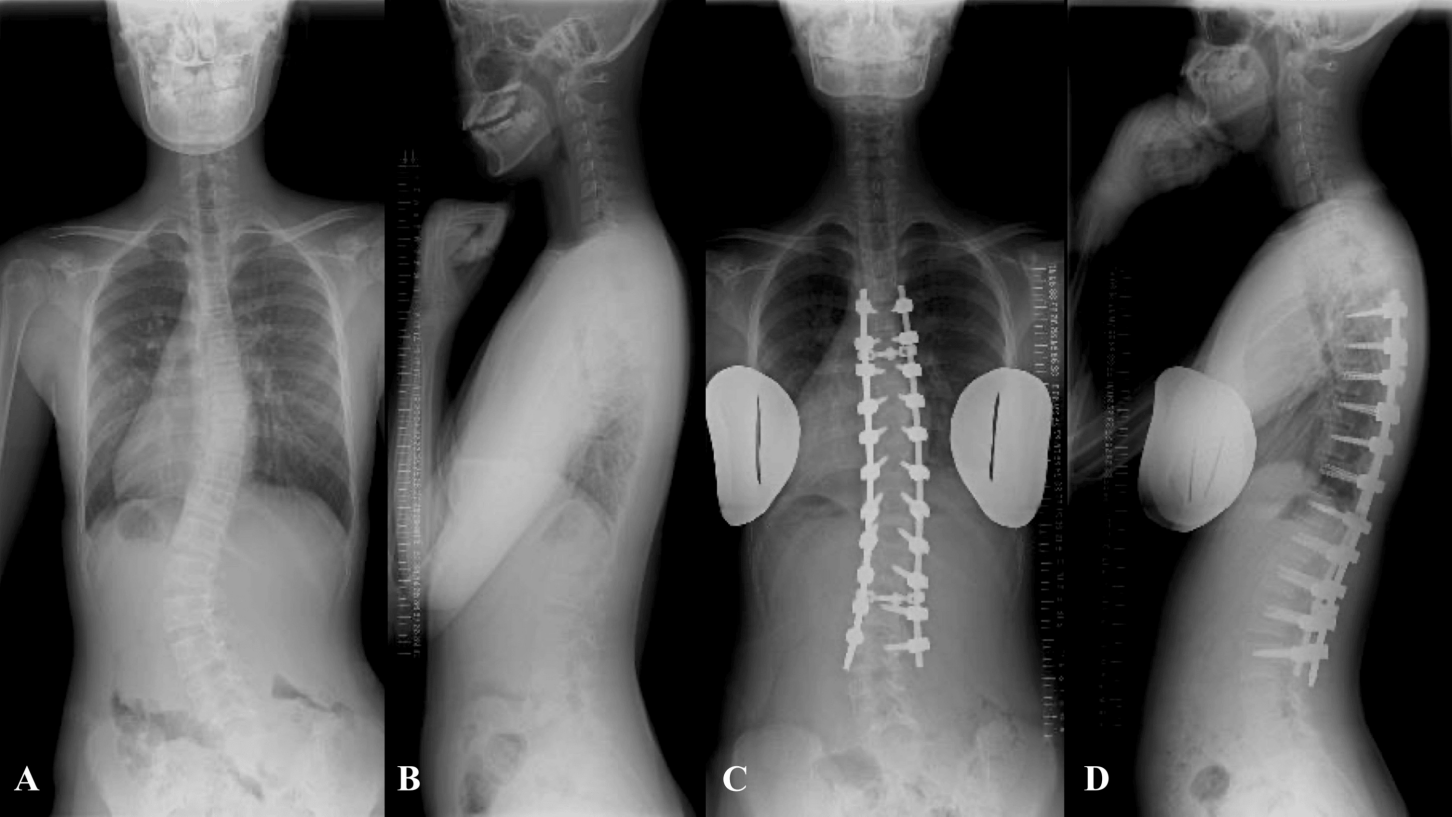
Case 1. Plain radiographs of the whole spine in a standing position. A: preoperative AP view, B: preoperative lateral view, C: postoperative AP view, and D: postoperative lateral view. AP: anteroposterior
